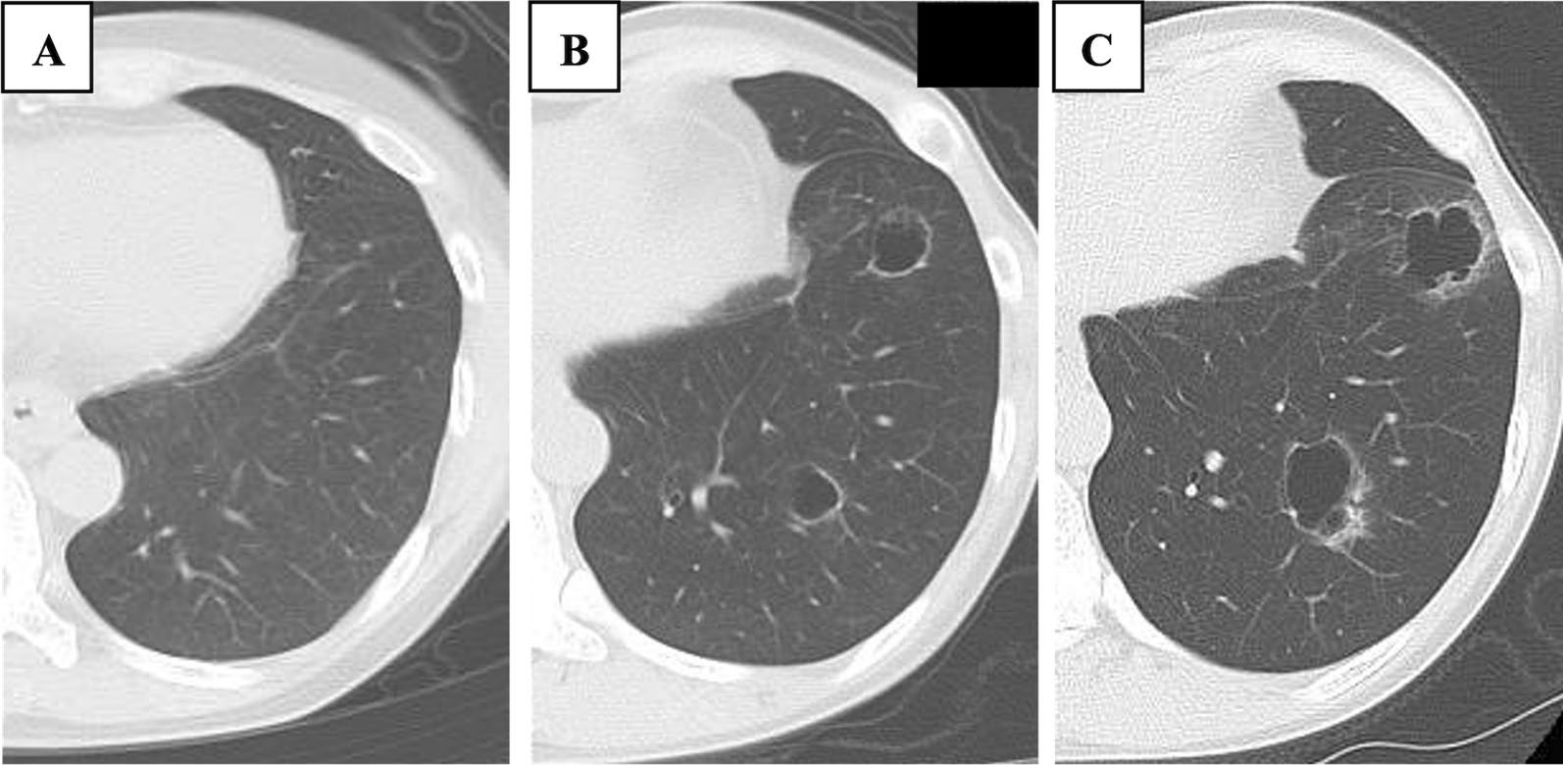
Changes on plain chest computed tomography. The cysts gradually enlarged and their walls were thickened. A 2 years after the initial surgery, B 3 years after the initial surgery, and C 3 years and 6 months after the initial surgery
